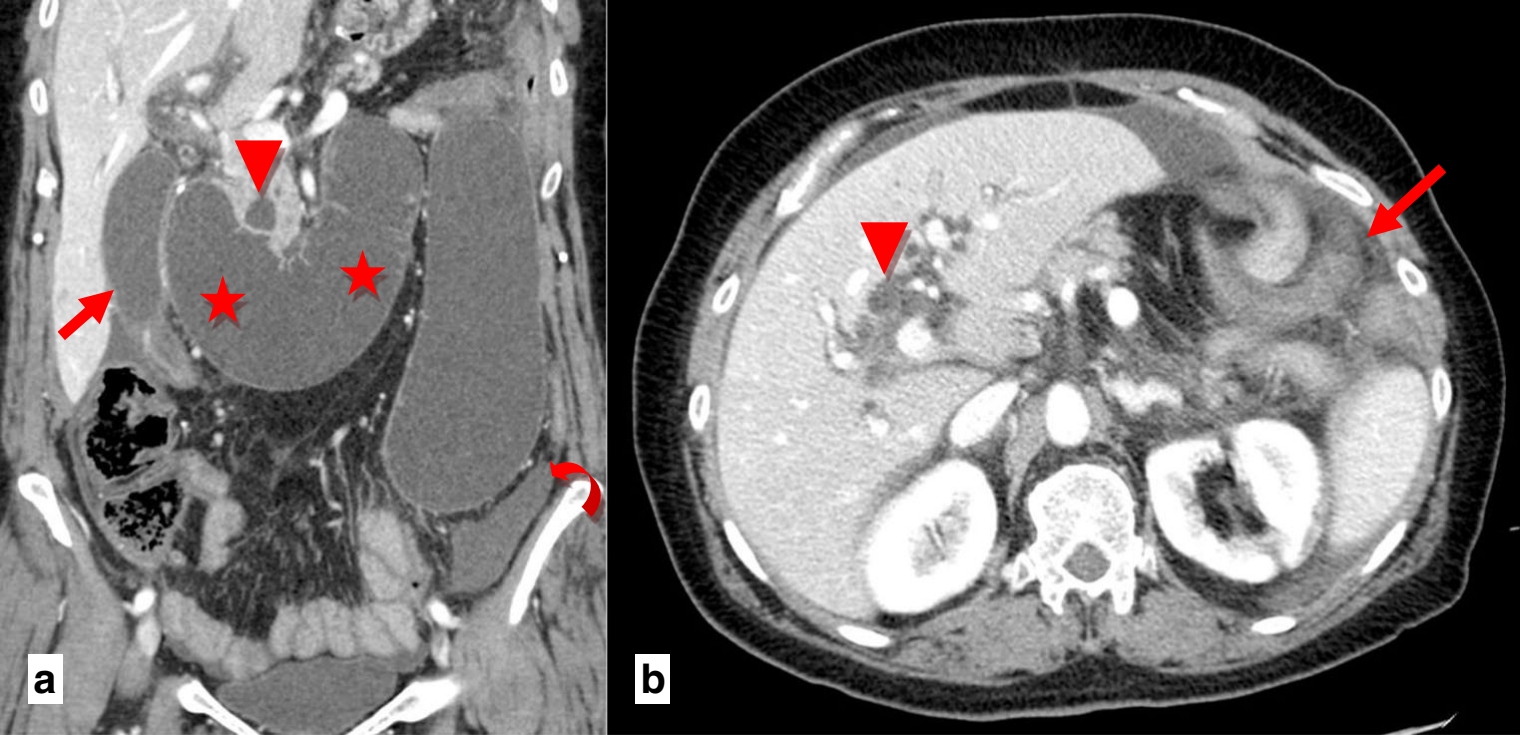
a, b Abdominal CT of a patient with progressively worsening abdominal pain, nausea and vomiting who had undergone Billroth II partial gastrectomy for gastric carcinoma 5 years earlier. There is marked fluid-filled dilation of the duodenum (*stars in a*), associated with gallbladder distension (*arrow in a*) and intrahepatic and extrahepatic biliary dilation (*arrowheads in a, b*), caused by an internal hernia with volvulus as shown by the twisted configuration of the bowel loops at the left upper quadrant (*arrow in b*). The ascites (*curved arrow in a*) suggests supervened ischemia
